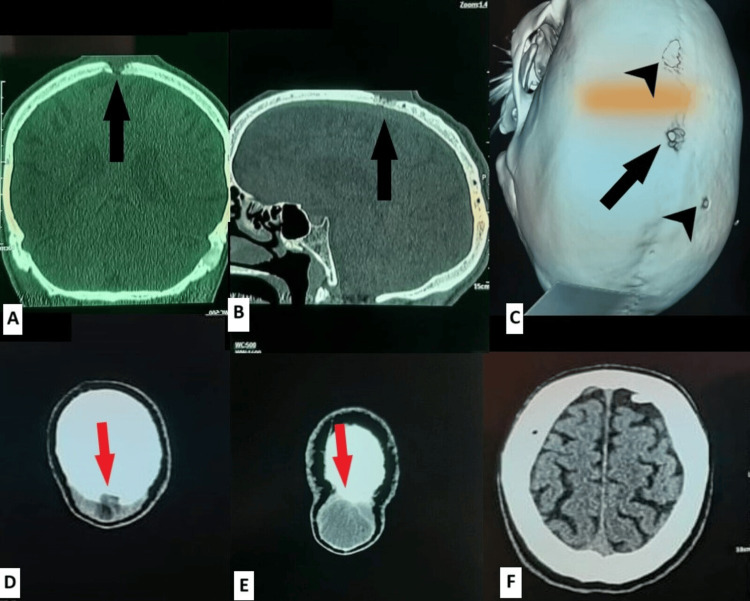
Preoperative CT scan images showing the midline mass. (A) CT scan of the head in coronal planes bone window showing a midline bone defect (black arrow). (B) CT scan of the head in sagittal planes bone window showing bone erosion in the parietal bone(black arrow). (C) 3D reconstruction of the preoperative CT scan showing a rounded bone defect at the parietal suture (black arrow) as well as bone defects (black arrowhead) at the left parietal bone and the right frontal bone. (D) and (E) CT scan of the head in axial planes parenchymal window showing a bulging cystic mass with extracranial and intracranial components (red arrow). (F) CT scan of the head in the axial planes parenchymal window showing the intact brain parenchyma with apparent signs of invasion or mass effect from the intracranial portion of the mass.
